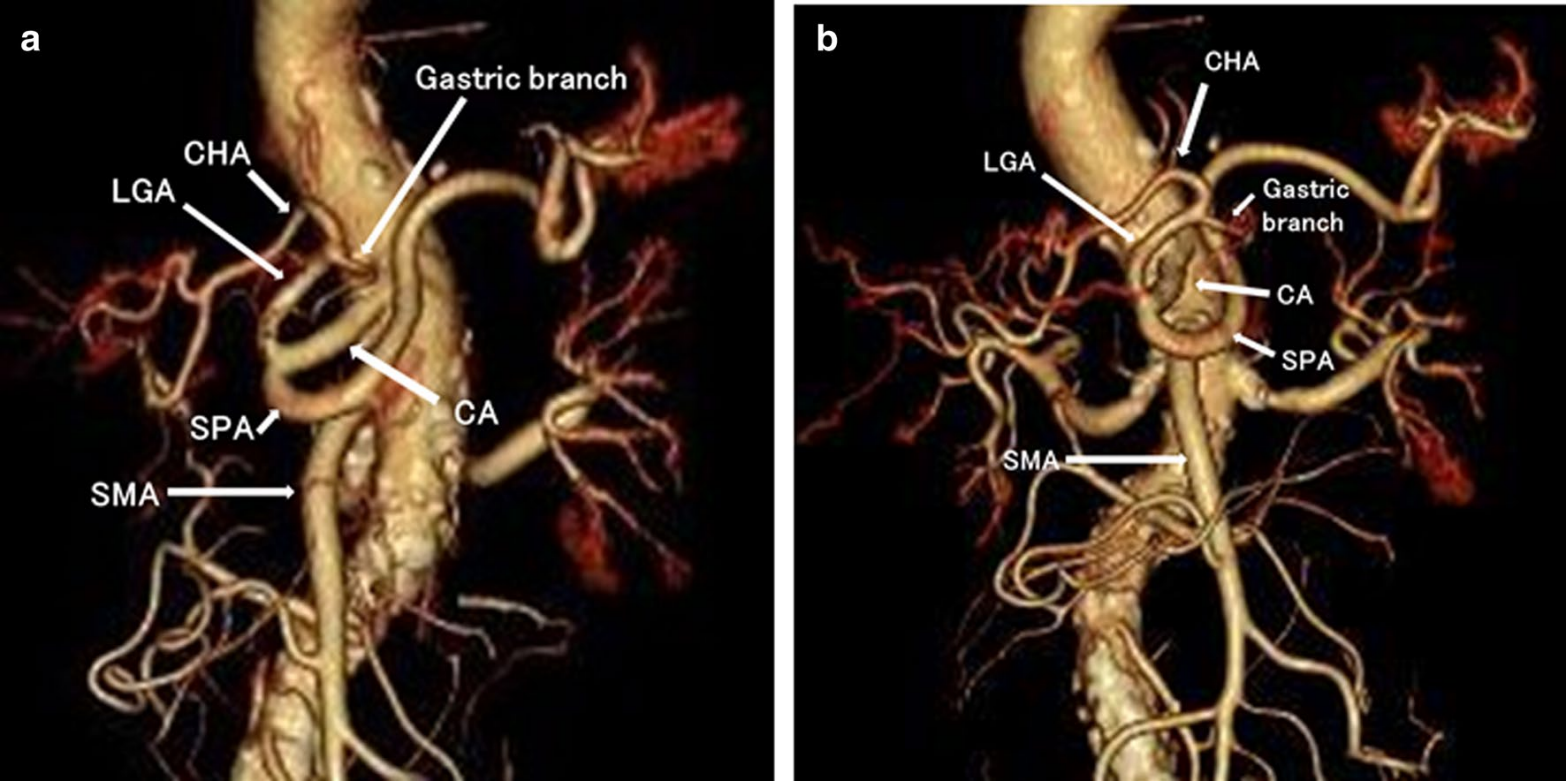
Enhanced computed tomography angiogram findings. Dynamic computed tomography angiogram showing the common hepatic artery branch from the left gastric artery. *CHA* common hepatic artery, *LGA* left gastric artery, *SPA* splenic artery, *SMA* superior mesenteric artery, *CA* celiac artery
